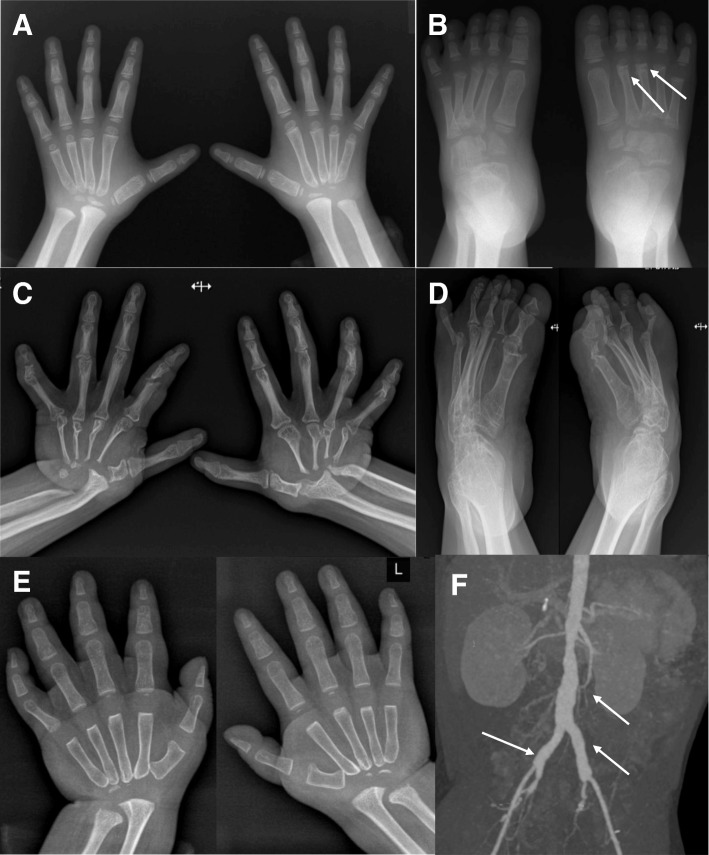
Radiological findings of 2 patients with multicentric carpotarsal osteolysis syndrome. In Patient 1, radiographs of the hands (a) and feet (b) obtained at 4 years old show severe bone resorption of the carpal and tarsal bones. Bone erosions are also noted in the proximal ends of metacarpal and metatarsal bones and the distal ends of the talus and calcaneus. Note that the distal metacarpal and metatarsal bones and the phalanges remained well-preserved at that time. Incidentally, there are fractures of the left 2nd and 3rd metatarsal necks (arrows). Follow-up radiographs of the hands (c) and feet (d) at the age of 20 show marked progression of bone resorption and associated joint contracture. In addition to the carpal and tarsal bones, the metacarpal and metatarsal bones, phalanges, distal radii, and ulnae are extensively involved. In Patient 3, radiographs of the hands (e) and feet (not shown) obtained at 1 year of age show multiple areas of osteolysis predominantly involving the carpal and tarsal bones. In addition, an abdominal aortic aneurysm was incidentally detected with renal ultrasonography (not shown), and a subsequently taken abdominal CT angiograph (f) confirmed fusiform aneurysms involving the infrarenal aorta and bilateral common iliac arteries (arrows)
